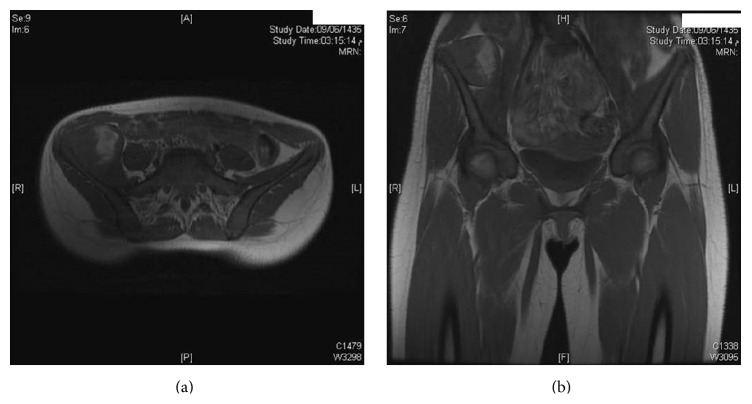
MRI images of the patient before initiation of neoadjuvant chemotherapy, showing mass arising along the right iliac bone, most probably soft tissue sarcoma with focal bony involvement.
